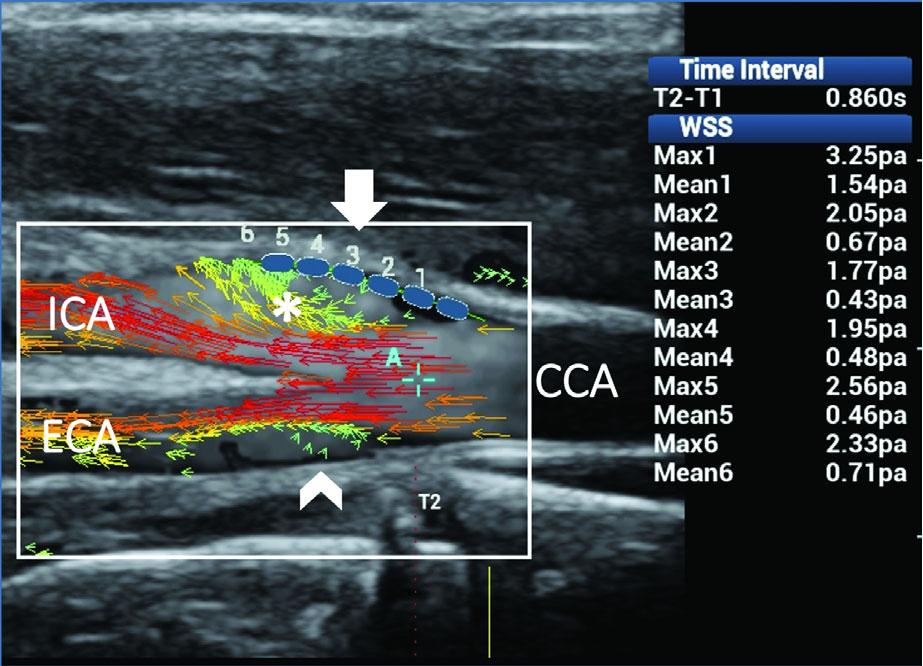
Rotational flow in the internal carotid artery (ICA). Ultrasound vector flow imaging shows flow layer separation in both the ICA sinus and external carotid artery (ECA). High‐velocity red vectors move along the 2 sides of the flow divider. Low‐velocity short green vectors rotate around an axis of flow along the outer wall while moving forward in the ICA (asterisk) and move back in a small recirculation area along the outer wall in the ECA (arrowhead). Wall shear stress measurements (blue dots) along the outer wall of the ICA (arrow) gave maximum and mean values within the normal range (1.77–3.25 and 0.43–1.54 Pa, respectively), thus confirming the steady shear stress related to the helical flow. CCA indicates common carotid artery.
